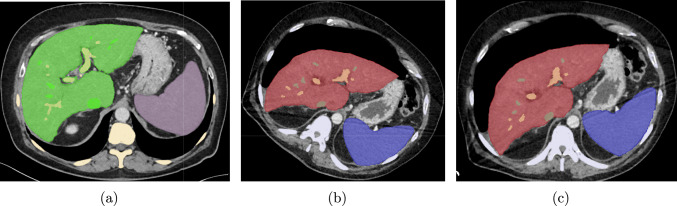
Axial slice of a a preoperative supine position CT image overlaid segmented labels (Green-liver, violet-spleen, yellow-spine, thick green-hepatic vein, and thick yellow-portal vein), b Intraoperative tilted position CT image with pneumoperitoneum overlaid liver parenchyma (red), spleen (blue), spine (gray), and hepatic vein (green), and portal vein (orange) segmentations, and c (Registered using spinal point) Transformed and resampled intraoperative CT image and its segmentations
